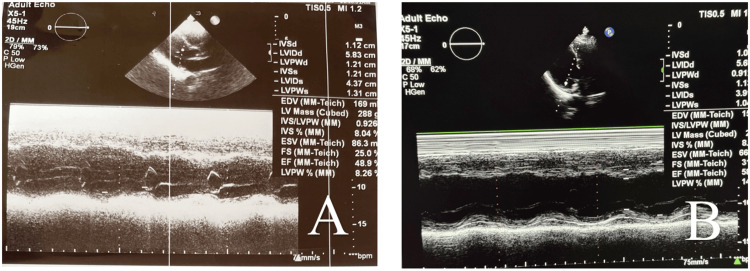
PLAX view of M-mode echocardiography (A) PLAX view on day 2 of admission showing a maximum ejection fraction of 48.9% after repeated measurements. (B) PLAX view at discharge showing improvement of ejection fraction to 58.3%, correlating with clinical recovery. PLAX: parasternal long-axis
